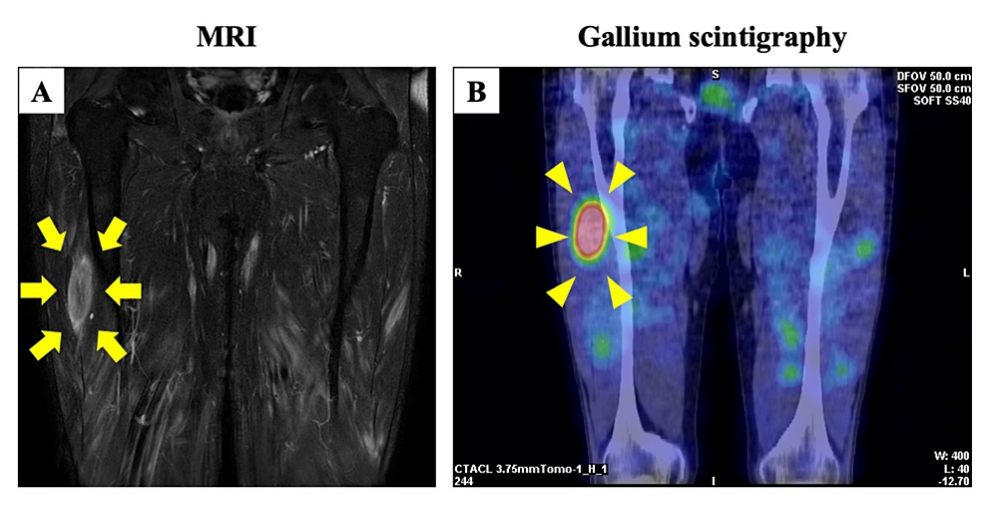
MRI (T2WI) and gallium scintigraphy (A) MRI (T2WI) shows the three stripe and dark star signs in the granuloma in the right thigh muscle layer (yellow arrow). (B) Gallium scintigraphy reveals accumulation in the same area (yellow arrow head).
